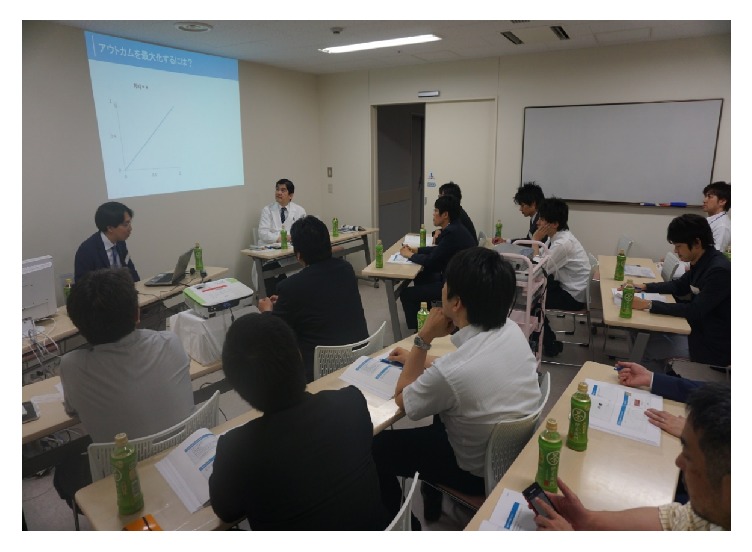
Lecture topics are current status of ablation, ablation systems, ultrasound systems, various techniques in ablation, and others.
